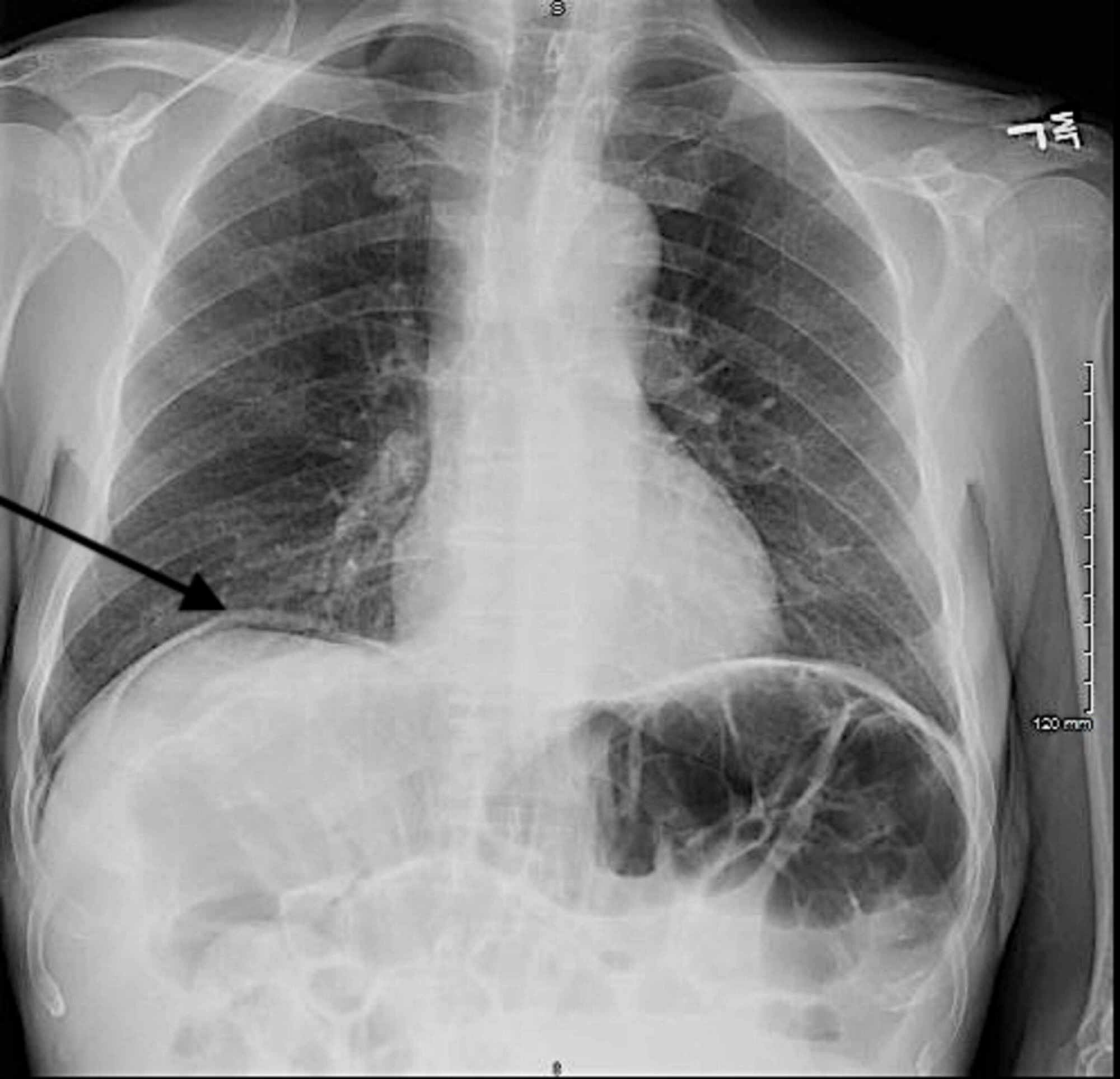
Portable chest radiography demonstrating free air under the diaphragm on the right (black arrow) consistent with bowel perforation.
